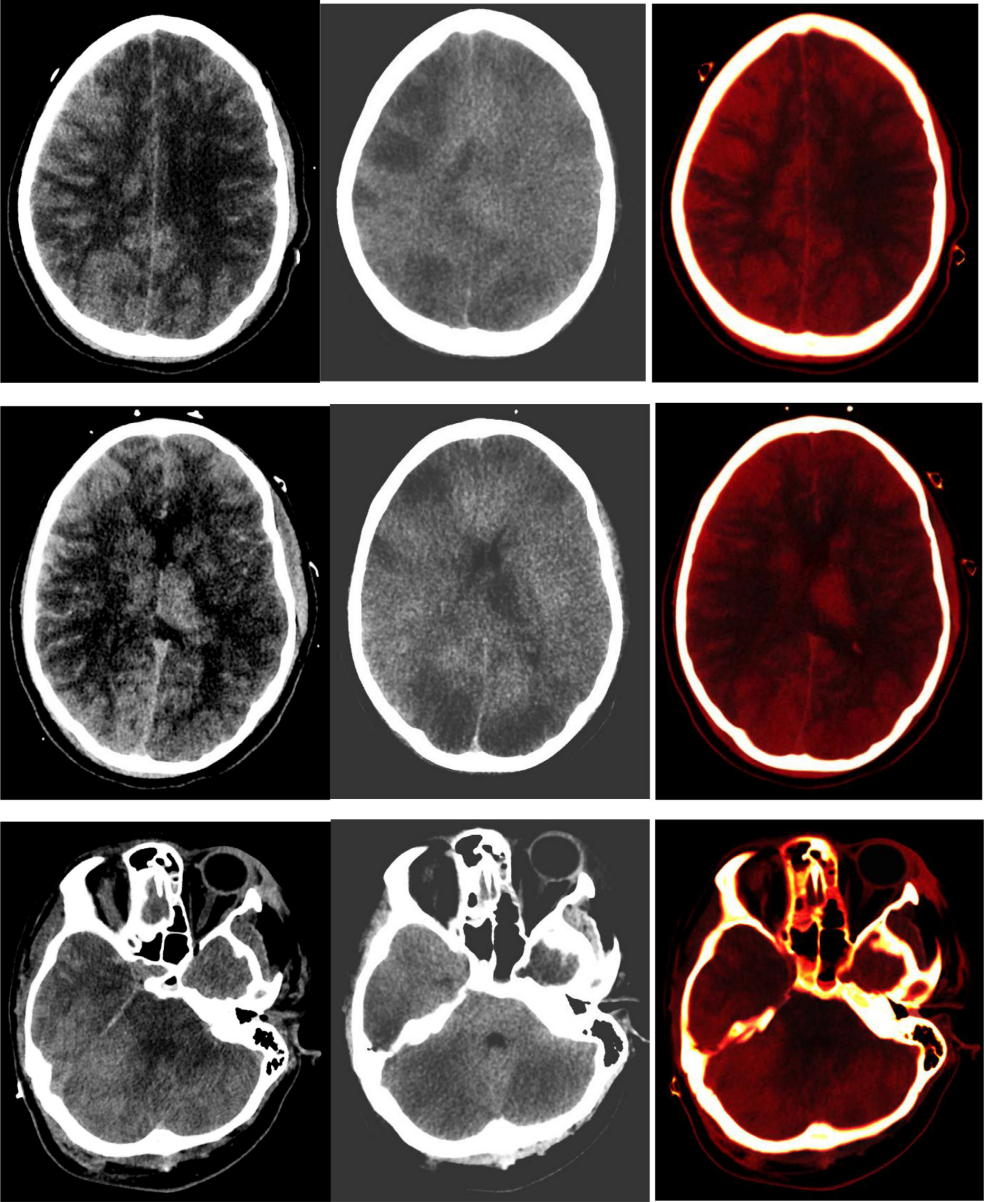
Left column: Non-contrast CT head demonstrates pronounced sulcal hyperdensity as well as hyperdense left thalamus, cortical and sub-cortical areas and cerebellum. Mean attenuation value was 103.17 HU in the right parasagittal parietal cortex and subcortical region, 109.25 Hounsfield unit (HU) in the left thalamus, and 112.27 HU in the right cerebellar hemisphere. Middle and right columns (Dual-Energy CT images): The right sided fused images of the brain demonstrate bright areas of contrast retention within the subarachnoid spaces as well as within patchy cortical and sub-cortical areas, left thalamus, and cerebellum. The middle subtracted virtual non-contrast (VNC) images demonstrate extensive low attenuation in areas of contrast retention consistent with widespread areas of infarction in a pattern consistent with diffuse hypoxic-ischemic injury.
